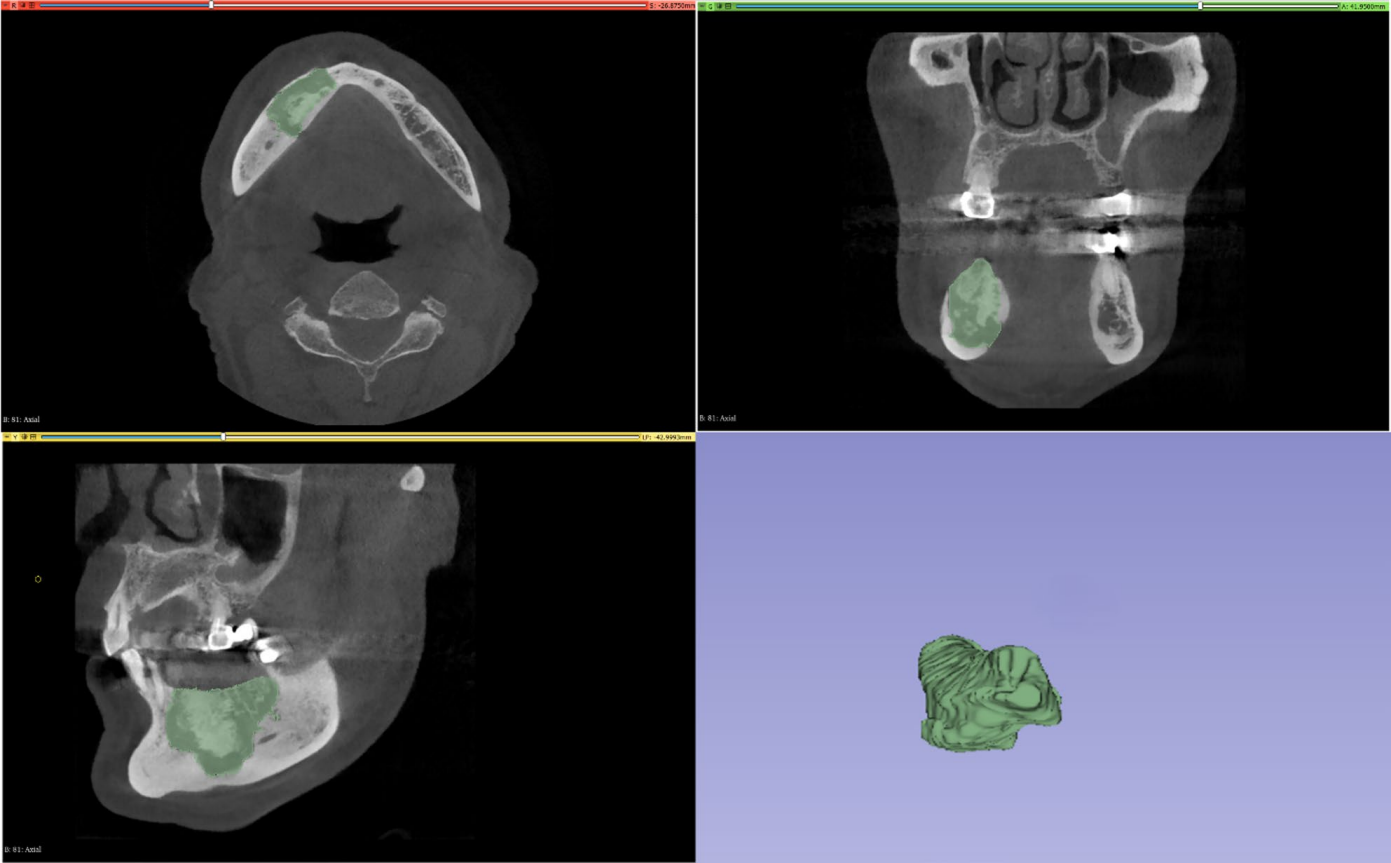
Labelling on axial, coronal and sagittal CBCT images and 3D model
